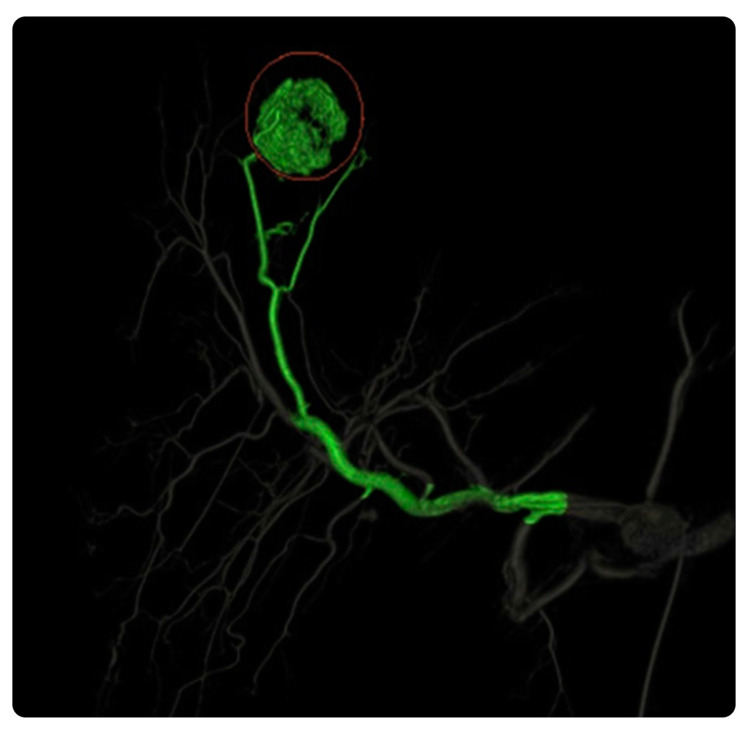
FlightPlan for Liver software and its role in intuitive display. FlightPlan for Liver enables the isolation of the relevant arterial vascular supply, automated vessel detection, and color coding (green) of arteries supplying the designated hypervascular region of interest. Reprinted from “Assessment of automated cone-beam CT vessel identification software during transarterial hepatic embolization: Radiation dose, contrast medium volume, processing time, and operator perspectives compared to digital subtraction angiography” by Durack et al. [78], Copyright 2018, with written approval from the corresponding author and publisher (Clinical Radiology. Copyright 2018).
